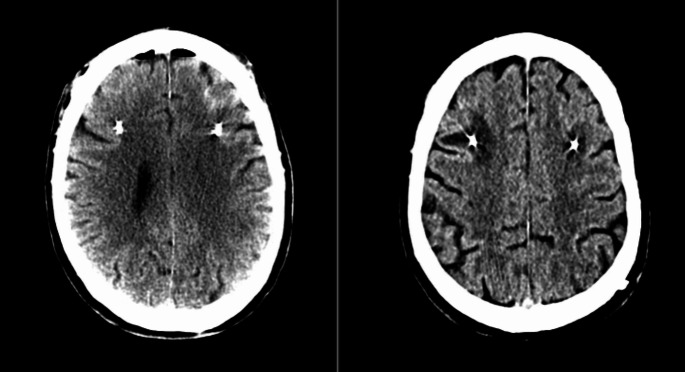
Postoperative same-day CT scan showing no edema (left) compared to a follow-up CT scan weeks later revealing the presence of edema (right)
